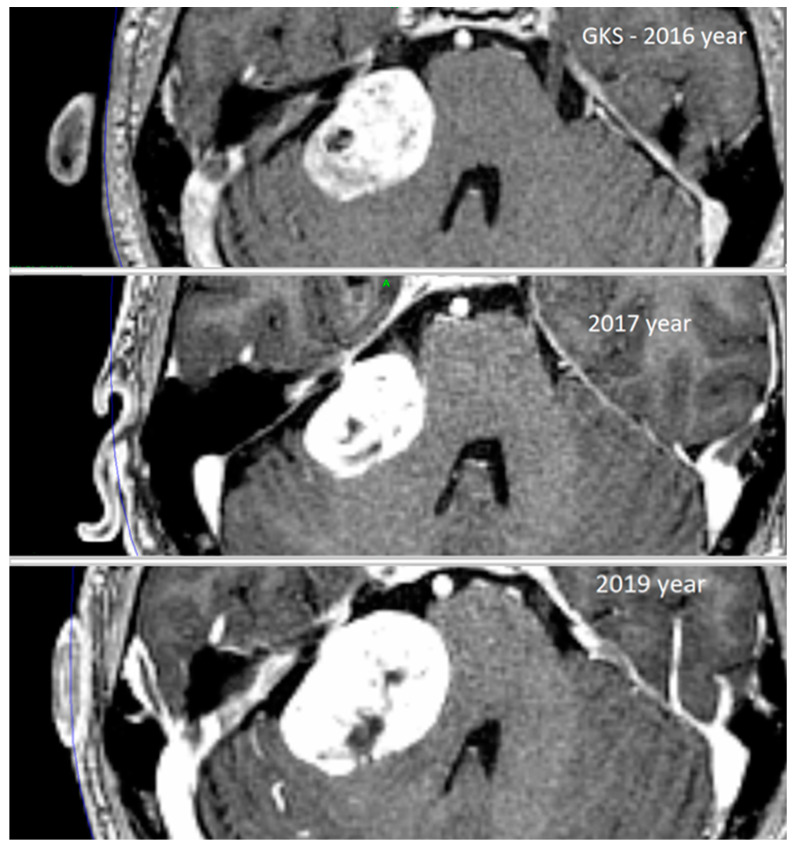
Patient No. 1. Enhanced magnetic resonance image showing the progression of right-sided giant vestibular schwannoma at the time of gamma knife surgery in 2016, a reduced tumor volume at the first follow-up visit in 2017 year, and progression of tumor volume at the last follow-up visit before surgical resection in 2019.
